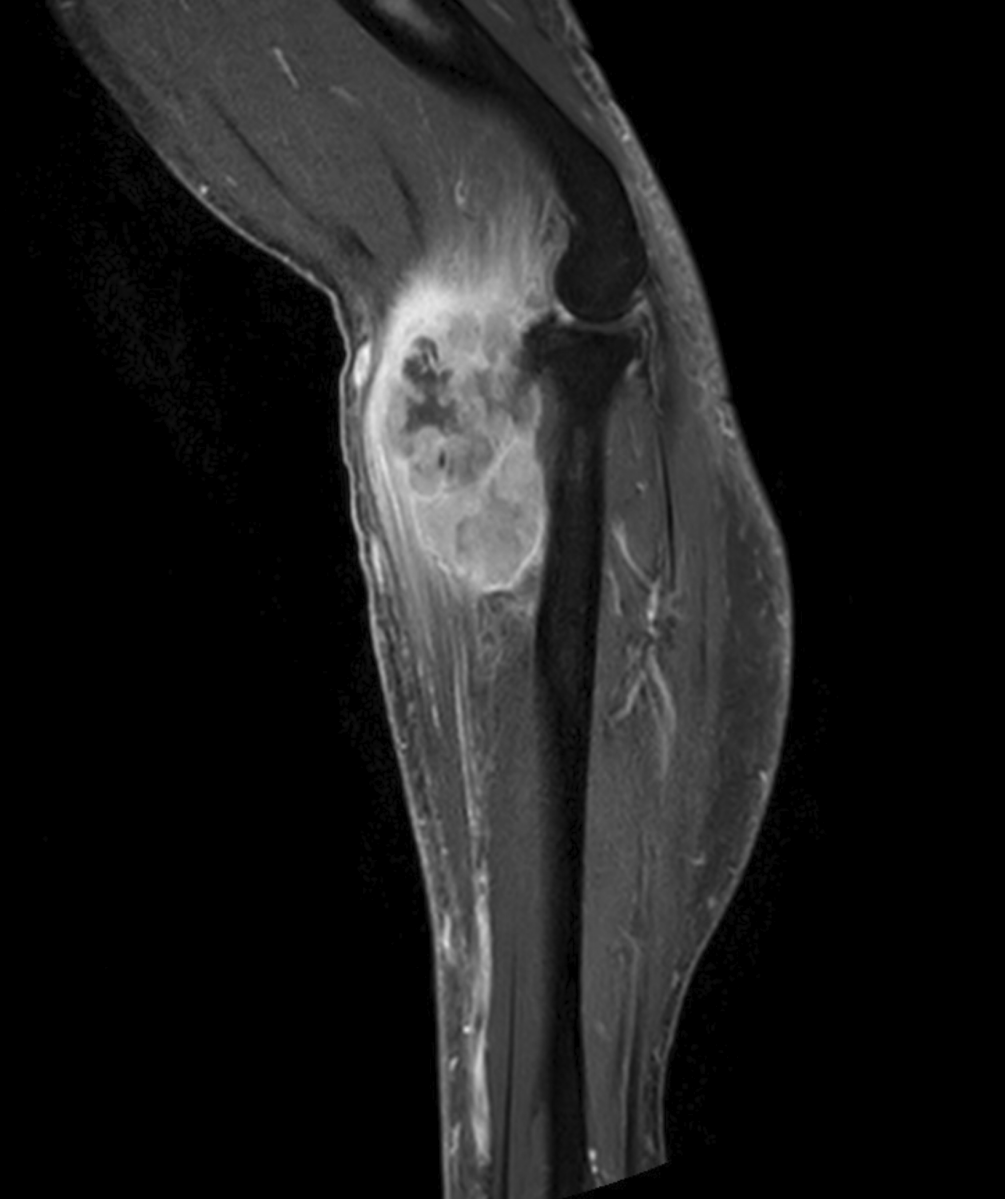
Magnetic resonance imaging findings of the right forearm. Massive lesion (9 cm × 4 cm) near the radius with suspected posterior interosseous nerve invasion.
